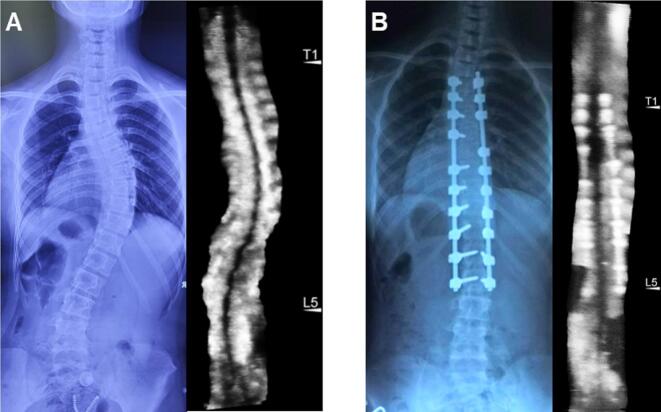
Comparative pre-operative and post-operative spinal ultrasound imaging in the same patient. (A) Pre-operative ultrasound scan. (B) Post-operative ultrasound scan.
